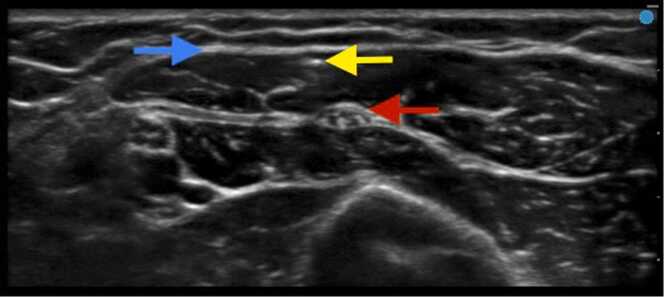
Cross-section ultrasound image of the hyperechoic implant (yellow arrow) within the biceps brachii muscle (fascia depicted as blue arrow) in close proximity to the musculocutaneous nerve (red arrow) and axillary sheath. Depth markers are seen on the right side of the image (total depth of the ultrasound image is approximately 2.3 cm).
